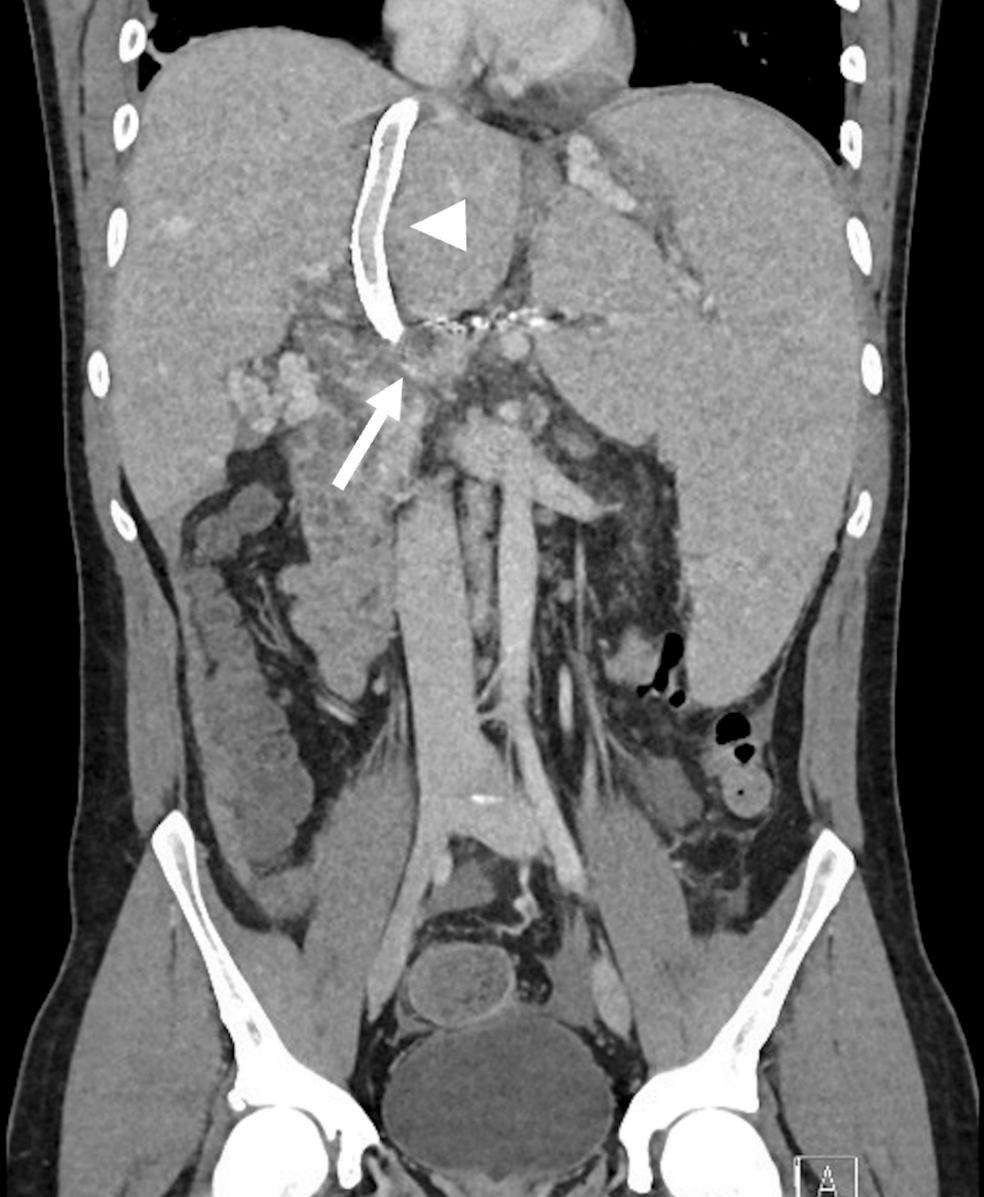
CT scan prior to discharge showing a patent TIPS (white arrowhead) and a reduced cavernoma (white arrow). TIPS: transjugular intrahepatic portosystemic shunt
